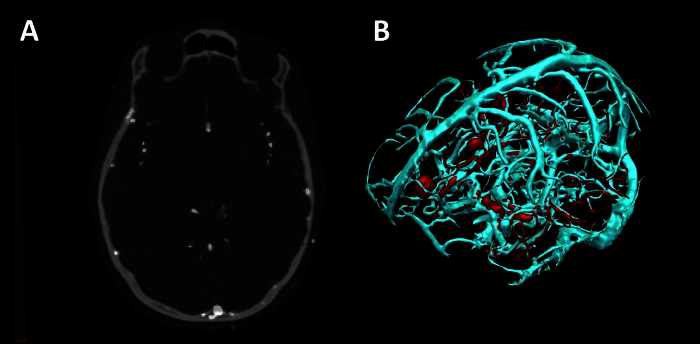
Figure 4. Vessel Extraction in In-house Software using Vesselness. (A) Axial CT angiogram co-registered with 3D phase contrast MRI. (B) 3D surface rendering of veins (cyan) and arteries (red). Please click here to view a larger version of this figure.
